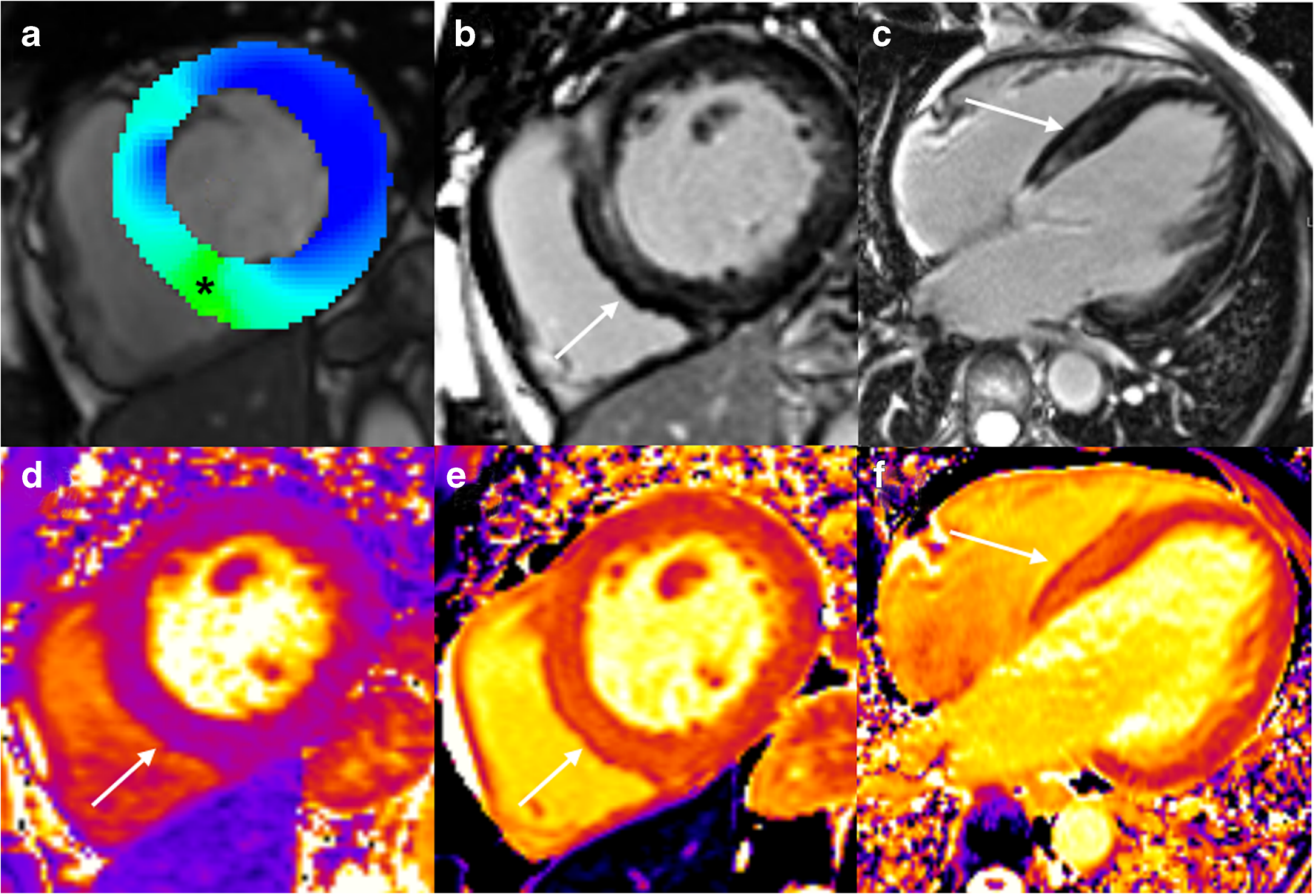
A 49-year-old male patient. New onset of dyspnea NYHA III since a SARS-CoV-2 infection 5 months ago. Cardiac MRI at 3T showing a dilated, eccentrically hypertrophied left ventricle (LV-EDV 151 ml/m^2^, LV mass 127 g/m^2^). Left ventricular (LV) ejection fraction (LV-EF 37%), LV peak circumferential (−12%), radial (18%), and longitudinal strain (−14%) were globally reduced, while the lowest regional peak circumferential strain was found in the LV septum (−6%, asterisk, a). Mid-ventricular Late-Gadolinium-Enhancement (LGE, b, c) was present in the LV septum, with normal T2 relaxation time (40 ms, d) in T2 mapping, but prolonged T1 relaxation time (1330 ms, e, f), consistent with myocardial fibrosis (white arrows)
